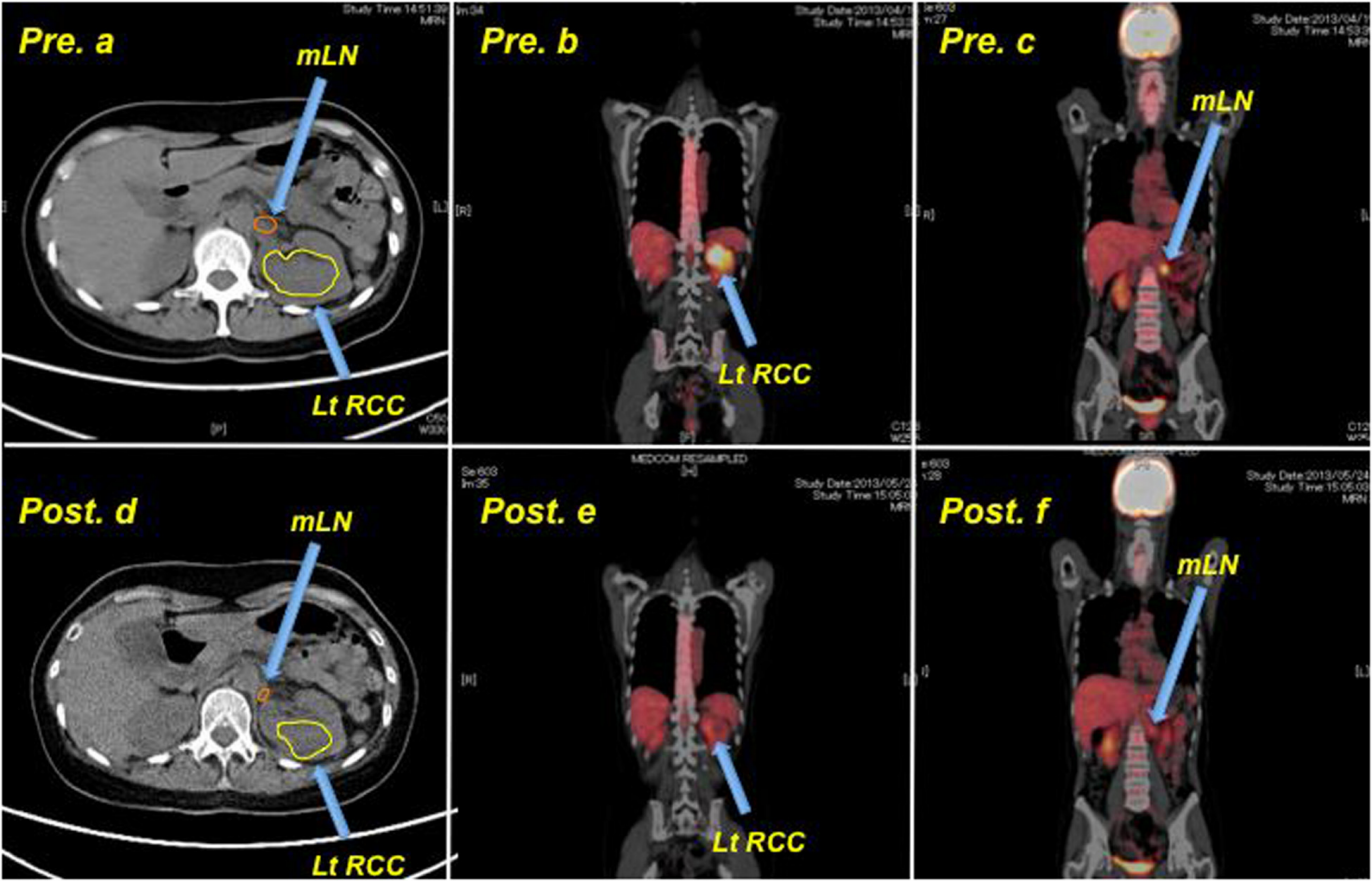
Positron emission tomography (PET) with [18 F] fluorodeoxyglucose (18 F-FDG PET) / plain computed tomography (CT). Pre: Before treatment with axitinib. Post: After administration of axitinib for 4 weeks. a, d: Plain abdominal CT shows that the primary left renal tumor and enlarged lymph nodes have decreased in size. b, e: SUVmax of the primary tumor decreased from 15.3 to 2.9 after administration of axitinib. c, f: SUVmax of the regional lymph nodes decreased from 7.5 to 2.3 after administration of axitinib
